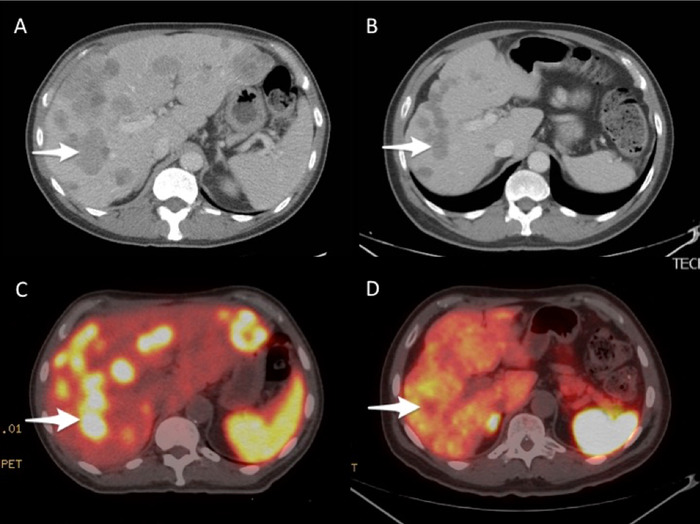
(A) Axial pretreatment contrast-enhanced computed tomography (CT) at the level of the right portal vein demonstrates innumerable hepatic metastatic lesions with index lesion (arrow). (B) Axial contrast-enhanced CT obtained 8 weeks after completion of peptide receptor radionuclide therapy (PRRT) demonstrates a decrease in the size of all lesions, including the index lesion (arrow). (C) Axial fused pretreatment gallium-68 dotatate positron emission tomography (PET)/CT scan demonstrates somatostatin receptor-rich hepatic metastatic lesions with index lesion (arrow). (D) Axial fused gallium-68 dotatate PET/CT scan obtained 8 weeks after completion of PRRT demonstrates resolution of tracer uptake within the metastatic lesions, including the index lesion (arrow).
